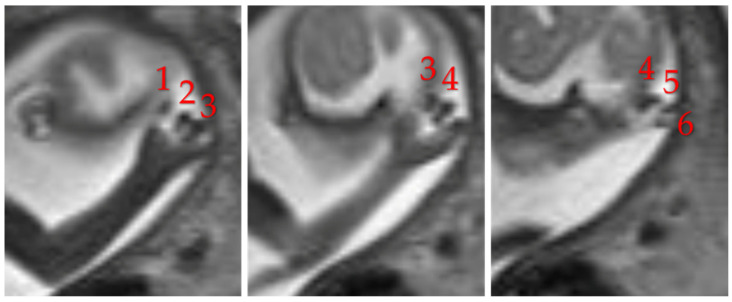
Fetal MRI T2W sequence at 21st week of gestation shows post-axial polydactyly (digits labeled with number).
